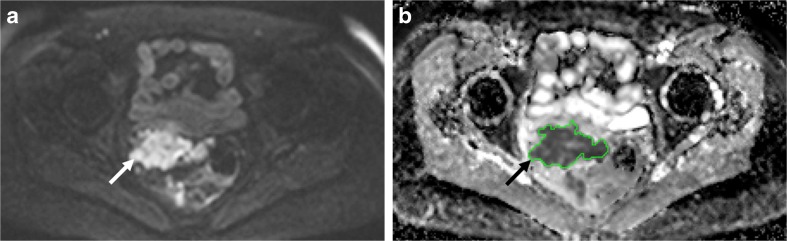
Diffusion-weighted-MRI in relapsed peritoneal cancer: Axial b-value=900 mm^2^/s (A) and image through the mid pelvis showing an irregular mass (arrow), with restricted diffusion contoured using a semi-automated region-growing tool. The tumour shows relatively limited signal decay with increasing b-value on the apparent diffusion coefficient map (B), and appears dark compared to normal tissues (arrow)
